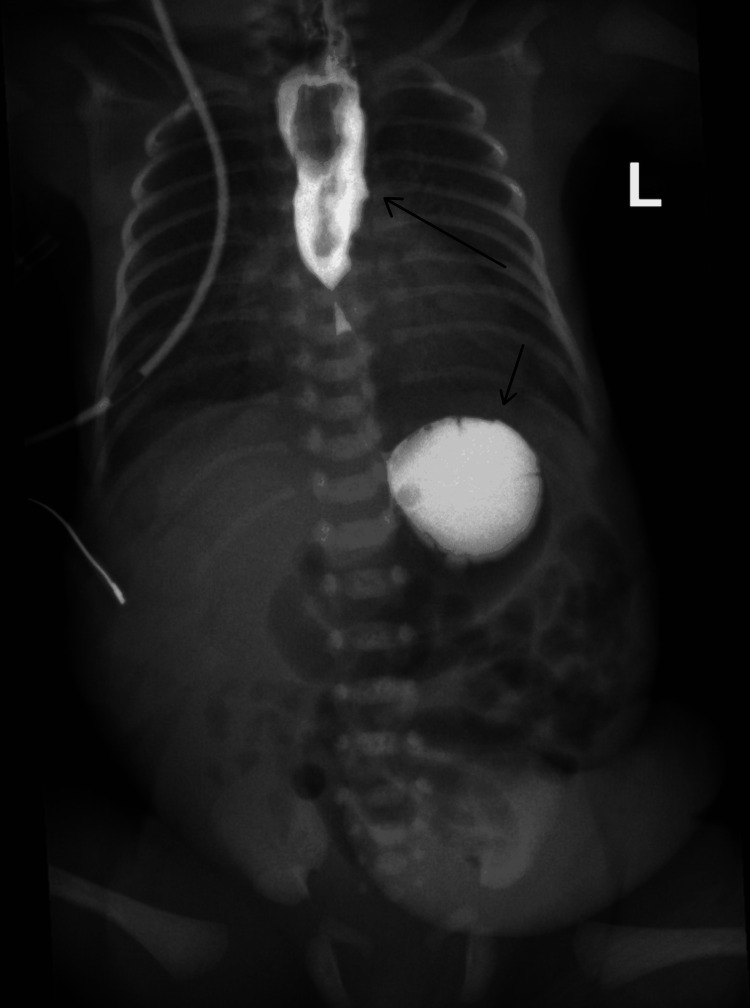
Contrast-enhanced X-ray showing contrast extravasation into the mediastinum causing a widened mediastinum X-ray with contrast injected through the OGT demonstrates proximal esophageal dilatation extending to the mid-esophagus (long arrow) and contrast material visualized within the gastric fundus (short arrow). OGT: Orogastric tube
